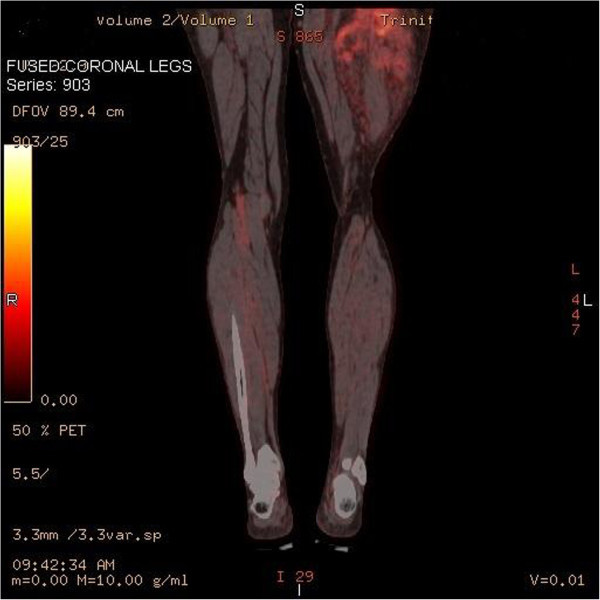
A coronal PET-IMG/CT demonstrating significant enlargement of soft tissue and fatty components in the biceps femoris muscle belly. Multiple serpiginous vessels are seen coursing through the soft tissue and surrounding edema.
